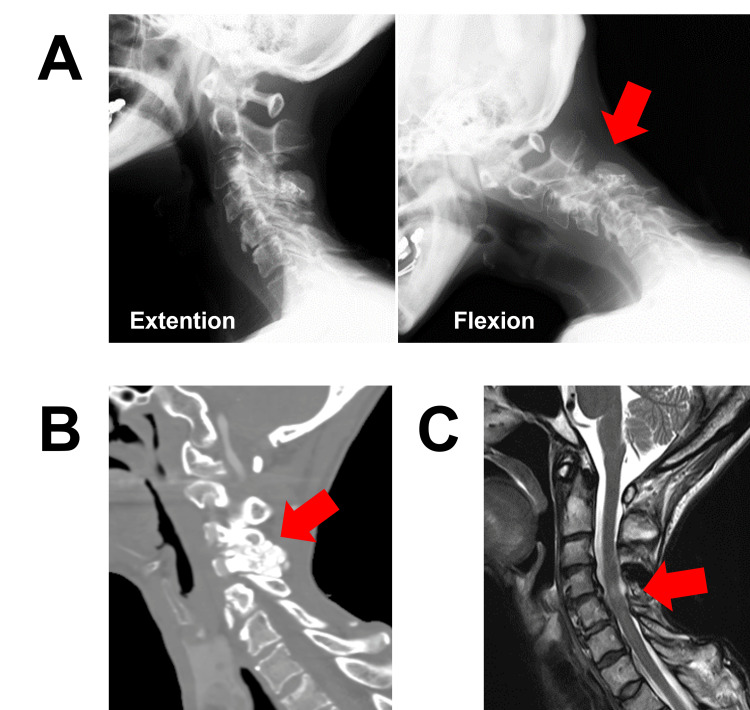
Preoperative images A) X-ray showed C4 anterior slip with dynamic instability. B) CT showed tumoral calcinosis of the connective tissues of the spine (ligamentum flavum and facet capsule). C) MRI showed intramedullary signal change at the C4/5 level.
